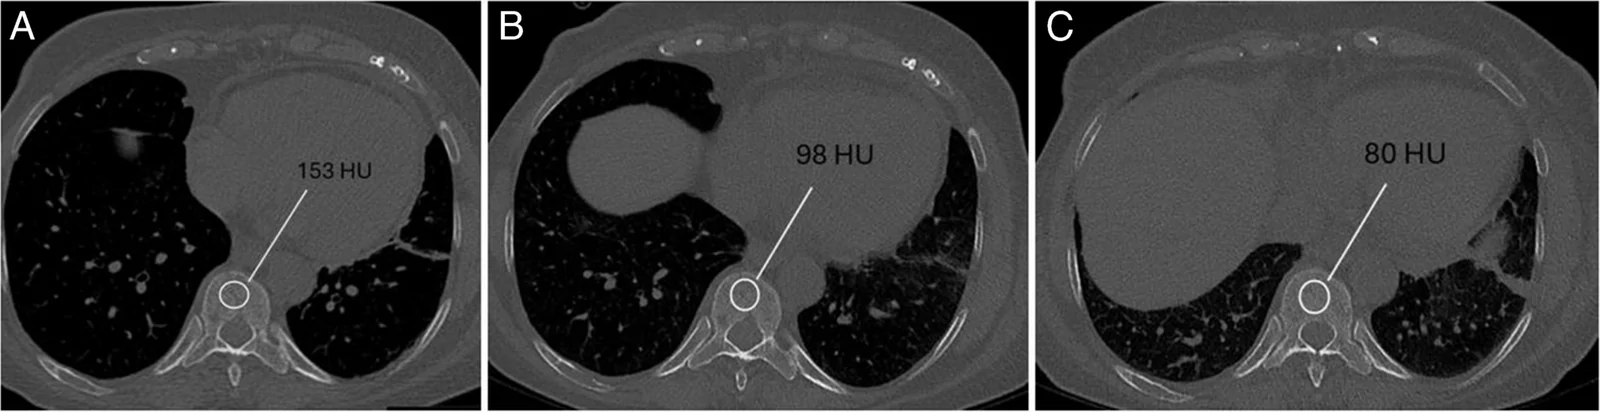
Representative axial non-contrast chest CT images demonstrating vertebral trabecular attenuation changes in a 76-year-old female patient undergoing autologous hematopoietic stem cell transplantation. (A) Pre-transplant CT shows normal trabecular attenuation (153 HU). (B) Early post-transplant CT at day 13 demonstrates a marked decrease in trabecular attenuation (98 HU). (C) Late post-transplant CT at day 134 shows further decline in attenuation (80 HU), consistent with progressive trabecular bone loss. Images are shown at slightly different axial levels because of variations in inspiratory volume and patient positioning between examinations. Vertebral attenuation measurements for study analyses were obtained from standardized ROI measurements at the mid-vertebral level of T10–T12 vertebral bodies, and mean values were used for analysis
